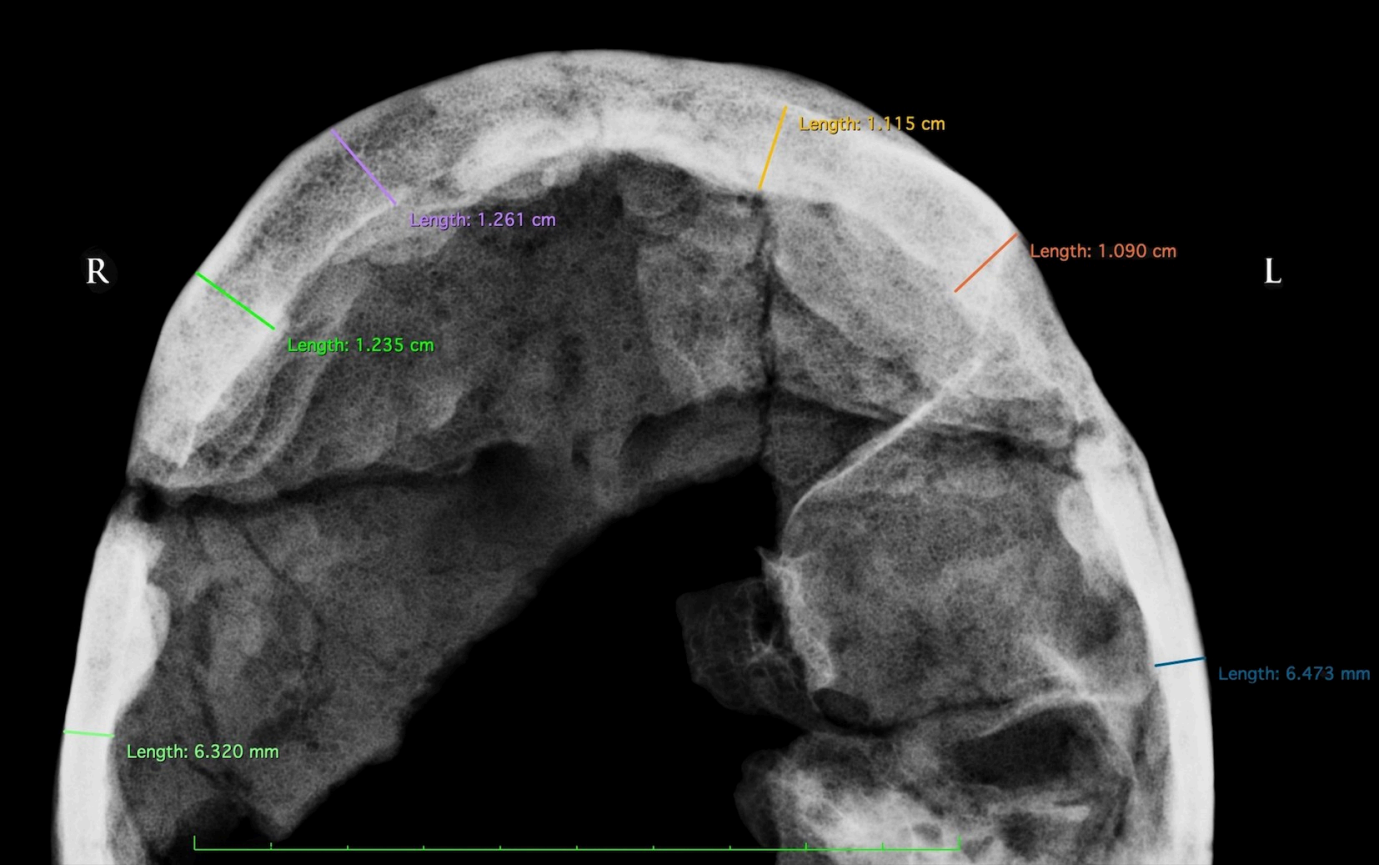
Postero-anterior X-ray with measurement of the thickness of the frontal and parietal bones indicating the hyperostosis. The obliteration of the frontal sinuses can be observed.
